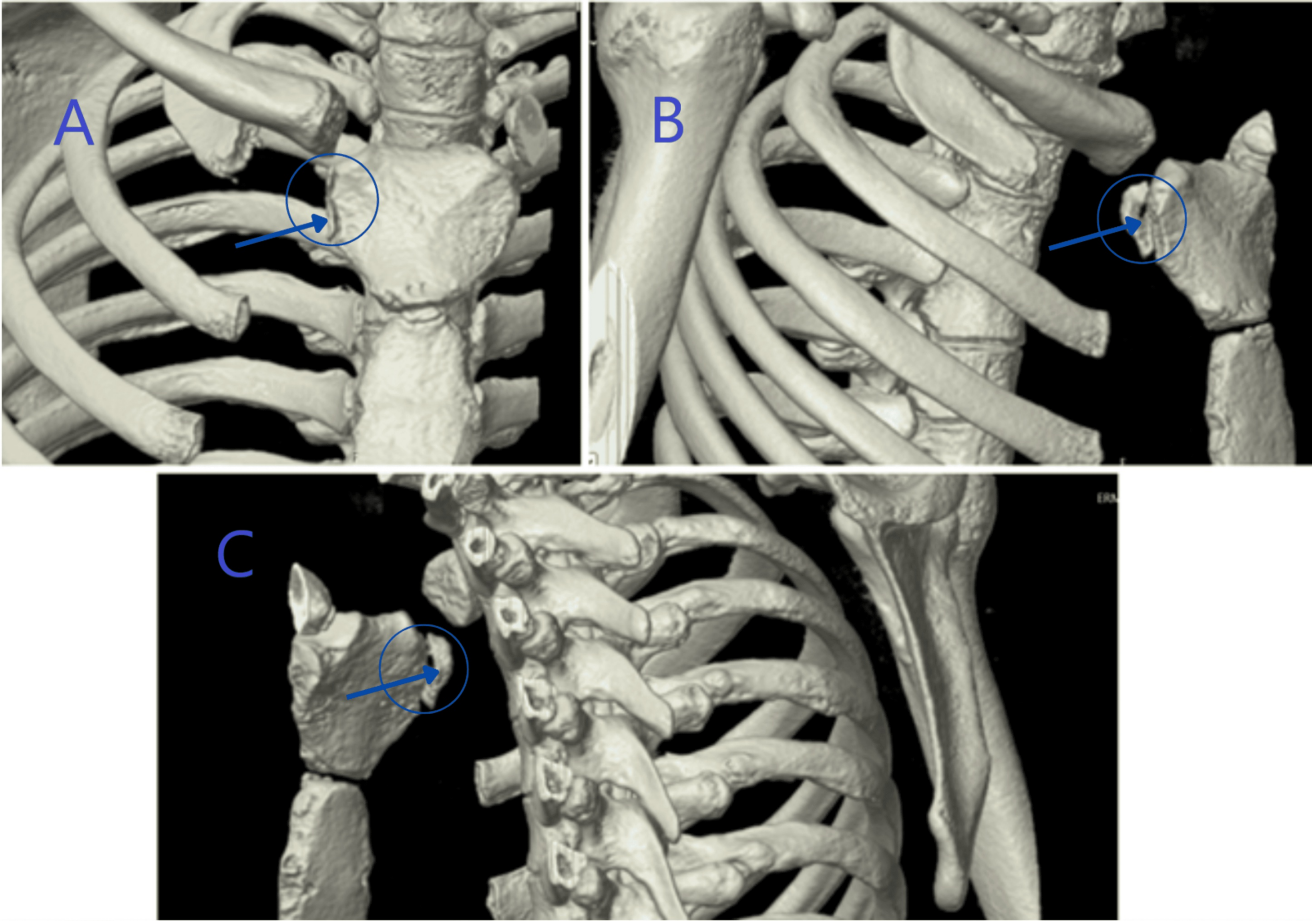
3D CT scans of the fractured manubrium A: coronal view highlighting the manubrium fracture (blue arrow); B: sagittal view highlighting the minimal displacement of the fracture (blue arrow); C: additional parasagittal view highlighting the minimal displacement of the fracture (blue arrow)
